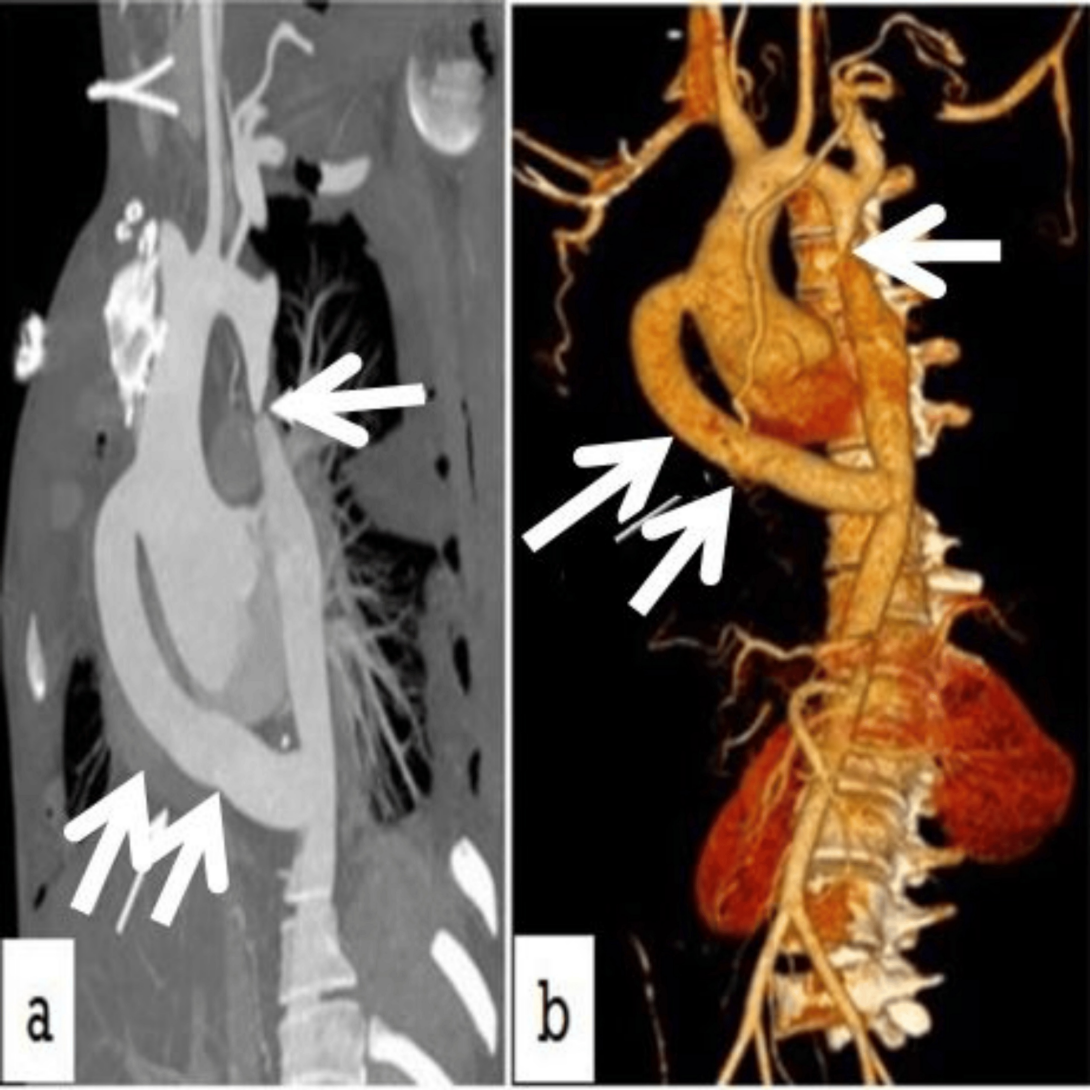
CT-aortogram findings a: postoperative CT-aortogram showing a well-opacified tube graft from the ascending aorta to the descending aorta with the native coarctation in situ (single arrow: juxtraductal segment of coarctation of the aorta; double arrows: Dacron tube graft); b: post-procedure three-dimensional reconstruction of CT aortogram (single arrow: juxtraductal segment of coarctation of the aorta; double arrows: Dacron tube graft)
